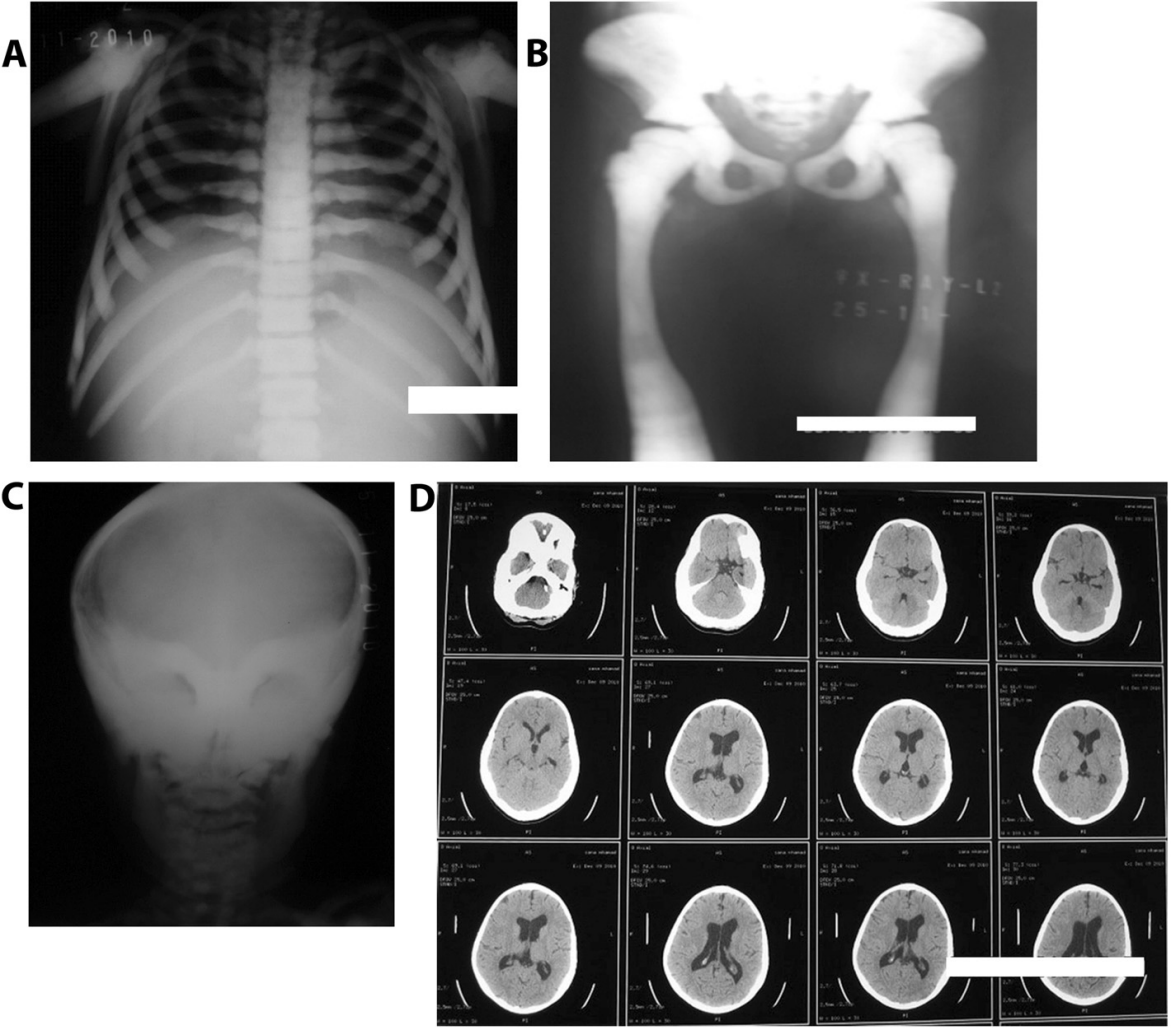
X-rays and computed tomography scan image of the patient showing bone thickening. Radiograph shows typical (A) vertebral endplate thickening (sandwich vertebrae sign) of the rib and vertebrae, (B) diffuse thickening of pelvic and lower limb bones, and (C) homogenous sclerotic skull bones. (D) A computed tomography scan image of the patient’s brain showing an increased thickened bone density, homogenously sclerotic skull bones encroaching on both optic foramens, and mild dilatation of the lateral and third ventricle with normal fourth ventricle.
